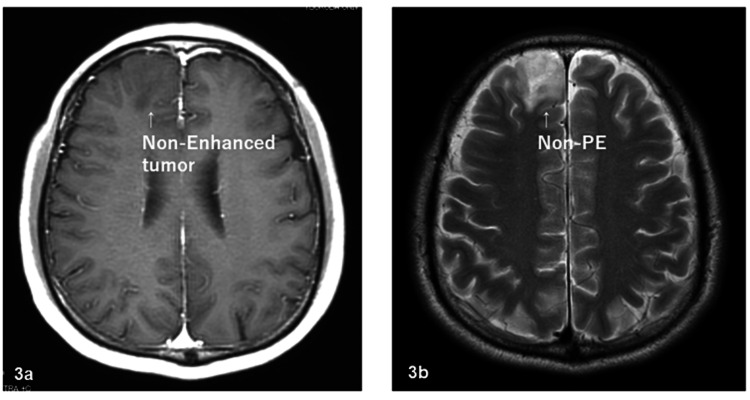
Supplementary MRI images – 3 3a: non-enhanced tumor before surgery; 3b: non-PE before surgery. The tumor shapes of 3a and 3b were almost the same MRI: magnetic resonance imaging; PE: preoperative edema
